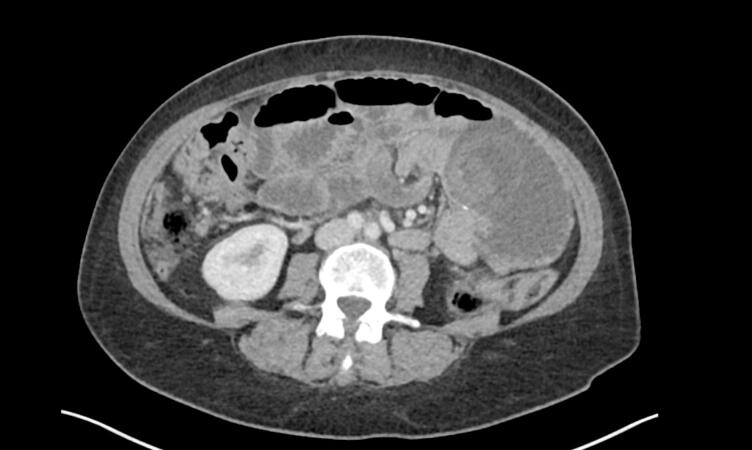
Axial slice of CT abdomen pelvis in the portal venous phase demonstrating high-grade small bowel obstruction with intussusception at the jejunojenunostomy site.
